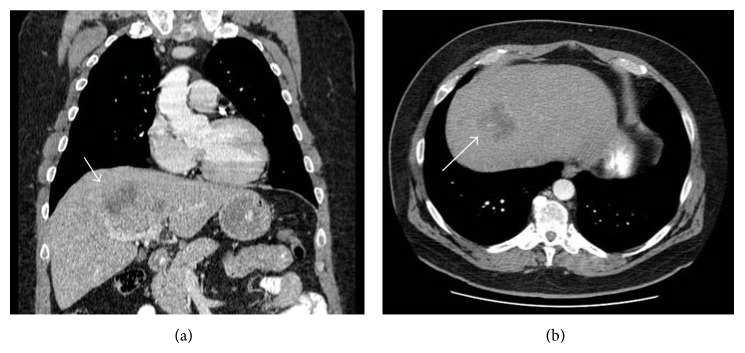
Abdominal CT scan showing hypodense lesion near the dome of the right hepatic lobe (arrow).
